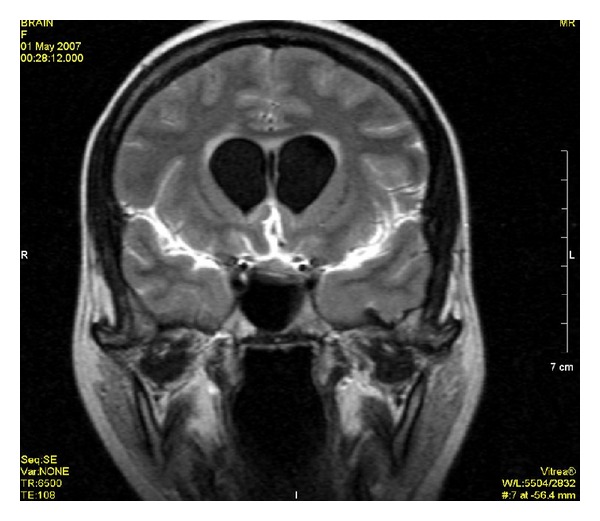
Postcontrast FLAIR image shows the enhancement of meninges at tentorium and in parietal region with evidence of dilated ventricles.
